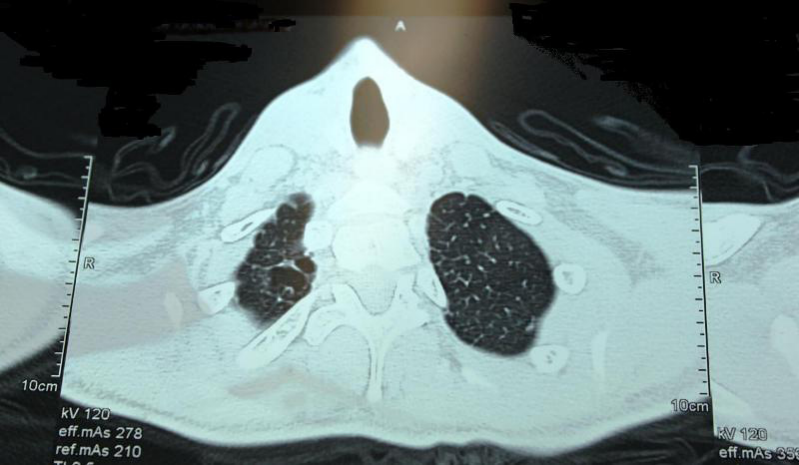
CT Image with the bullae of the right lung apex.
